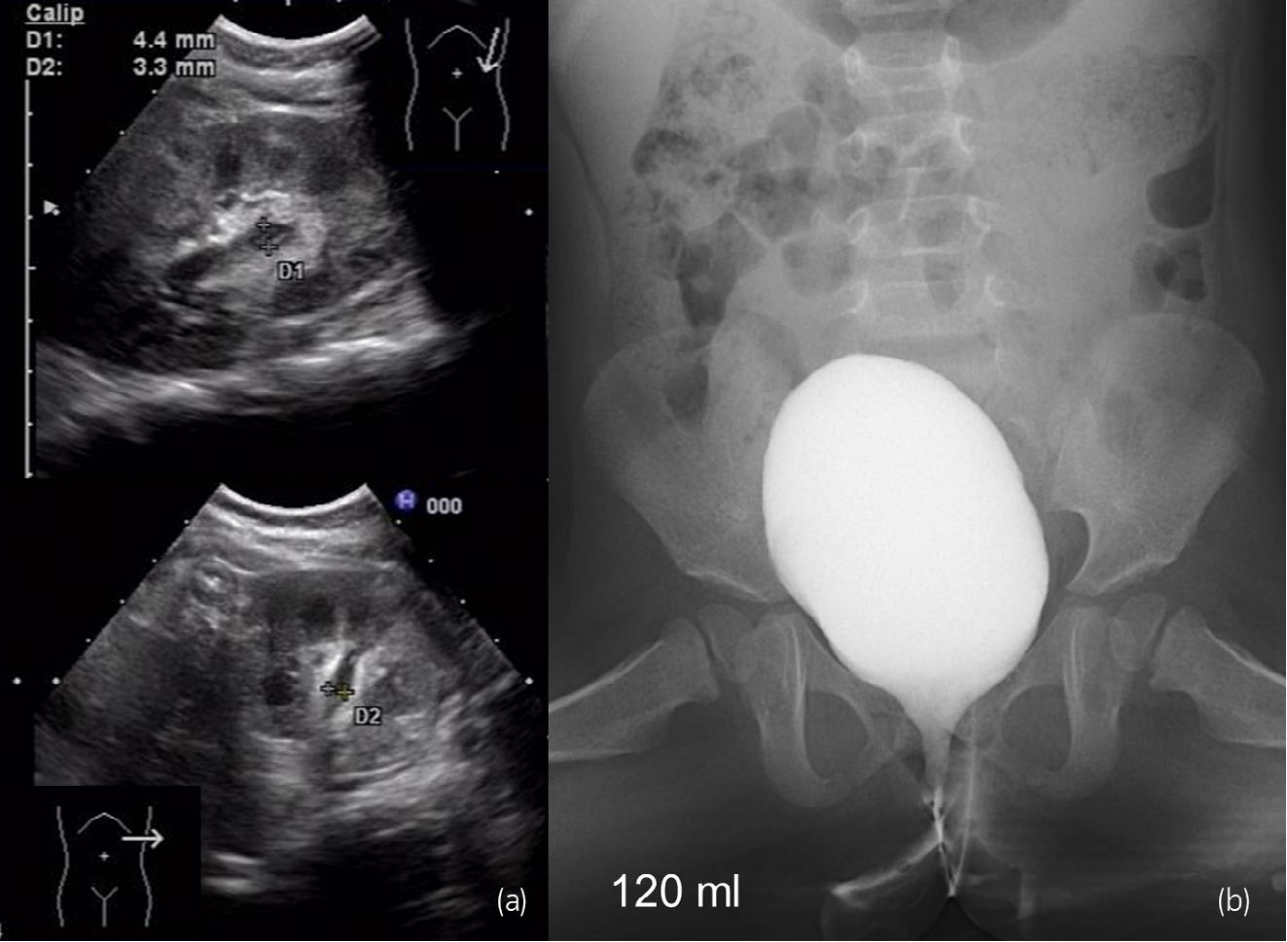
(a) Ultrasonographic images obtained at 2 years of age showing left‐sided hydronephrosis grade 1. (b) Voiding cystourethrography and cystometrometry performed at 2 years and 8 months show disappearance of VUR, improvement of bladder capacity to 120 mL, and improvement of bladder compliance to 13.3 mL/cmH_2_O.
